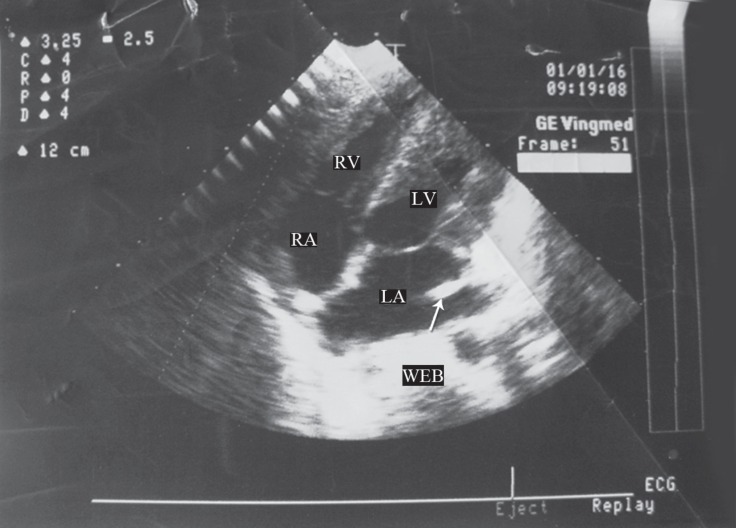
Four-chamber echocardiography view, showing the presence of the Eustachian valve and entrance of the inferior vena cava into the left atrium (arrow)
